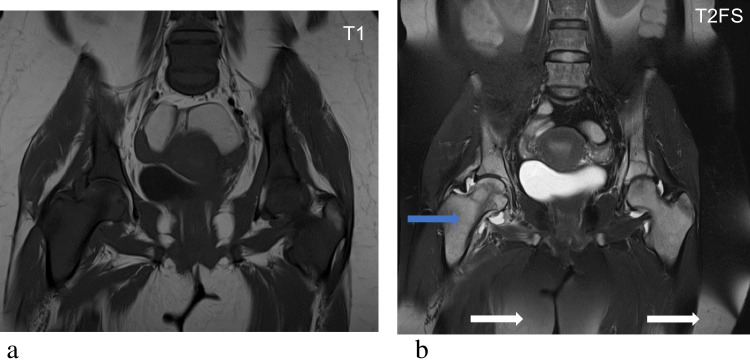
Acute leukaemia in a 32-year-old female with incomplete fat suppression on fluid sensitive sequences. Coronal T1-weighted image (a) of the pelvis, lumbar spine and proximal femora demonstrate diffuse hypointensity of bone marrow in keeping with marrow infiltration. Corresponding T2-fat saturated sequences (T2 FS) (b) demonstrate diffuse hyperintensity of bone marrow with serpiginous areas of signal abnormality in the femoral heads and adjacent acetabula, suspicious for osteonecrosis. There are also peripheral band-like signal changes that do not persist on the T1-weighted images, in keeping with incomplete fat saturation. Therefore, the T2 FS image mimics the inverted signal changes of SABM with hyperintense signal changes in the marrow (blue arrow) and subcutaneous tissues (white arrows)
